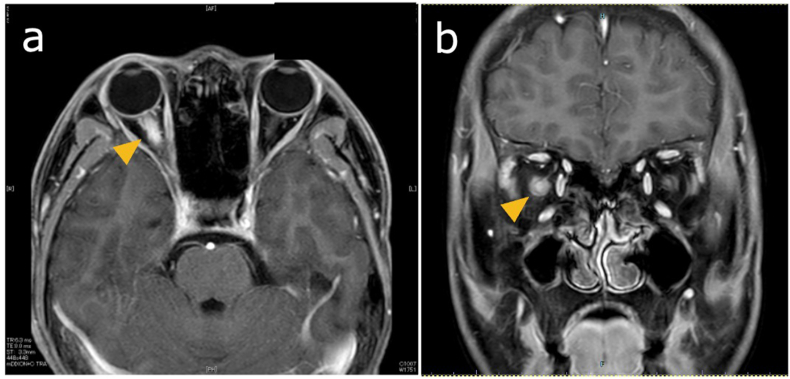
Magnetic resonance imaging (MRI) findings for a 14-year-old Japanese boy with acute macular neuroretinopathy associated with optic neuritis. MRI of the orbits shows swelling and enhancement of the right optic nerve and perineural space (a: axial, b: coronal).
